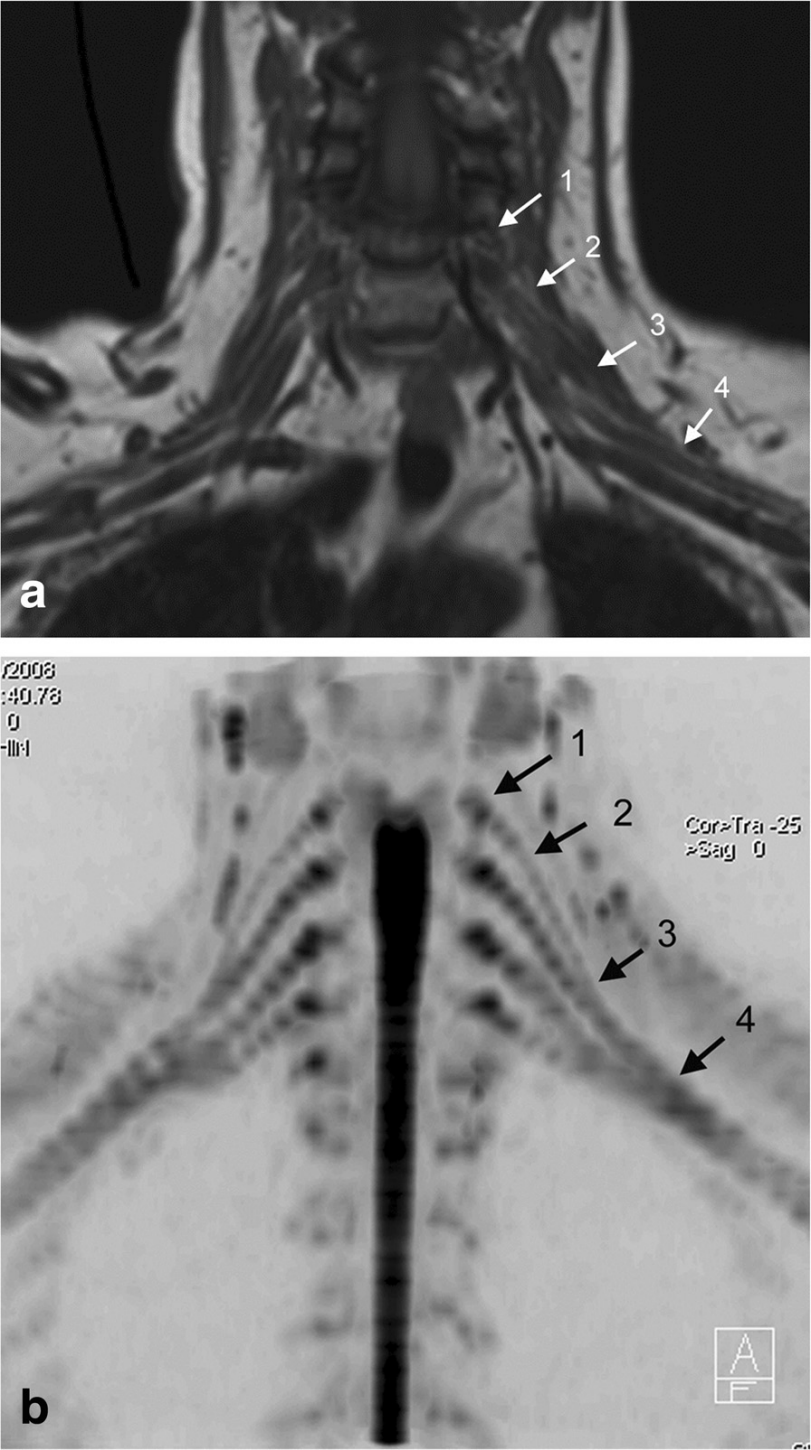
Anatomic evaluation of the brachial plexus on conventional imaging and DW-MRN. (a) Coronal T1-weighted image and (b) Inverted greyscale b = 700 s/mm^2^ coronal maximum intensity projection (MIP) from DW-MRN. The visibility of neural structures at the levels of the nerve ganglia, nerve roots, trunks, as well as divisions and cords (see arrows: 1 = ganglia, 2 = nerve roots, 3 = trunks, 4 = divisions and cords) were assessed visually.
